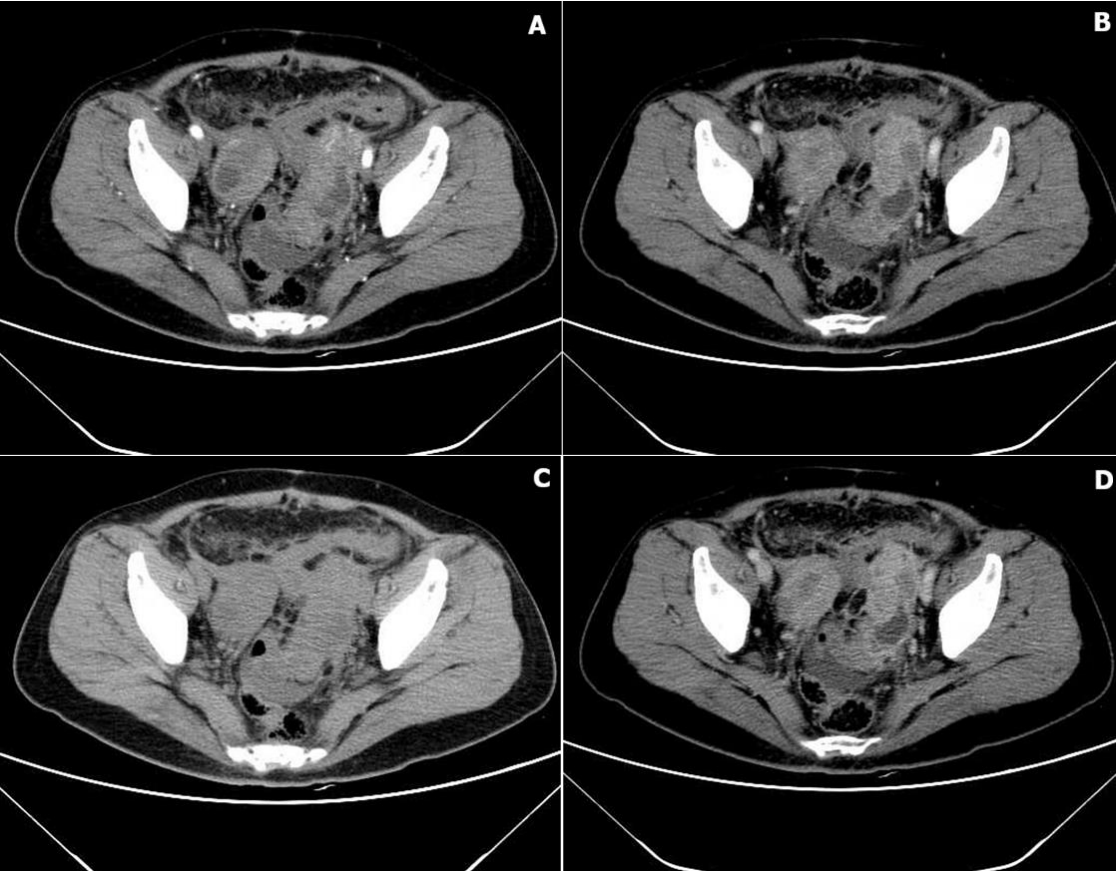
contrast CT: A) arterial: mild heterogeneous enhancement; B) venous: centripetal enhancement; C) unenhanced: mixed cystic-solid, lobulated, stranding; D) delayed: solid persists; cystic hypodense (*)
